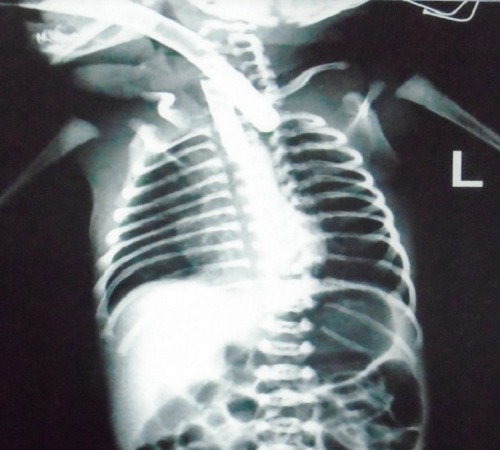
Figure 1: The radiograph revealed red rubber catheter in upper pouch and a cylindrical foreign body in the upper aero-digestive tract.
